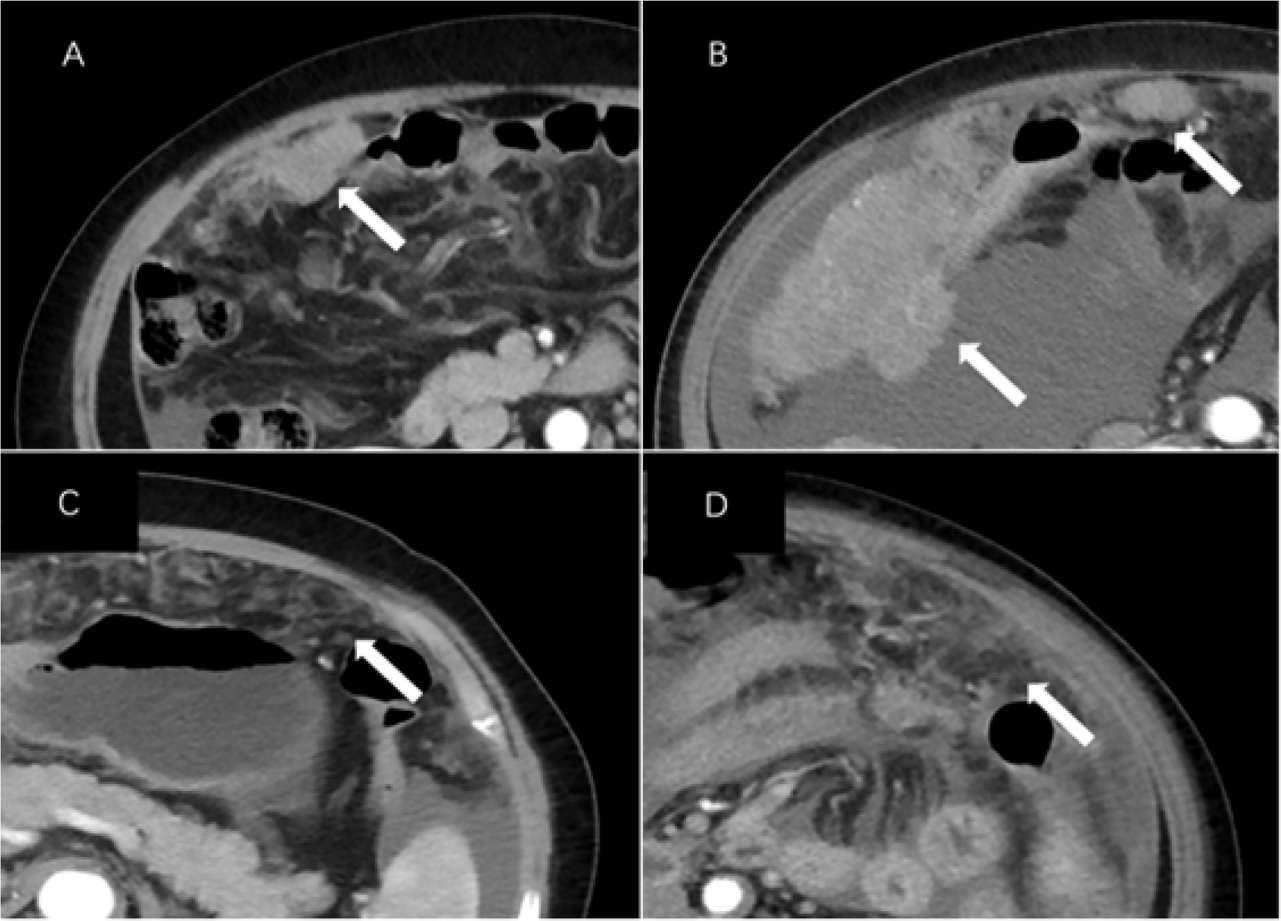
CT images of peritoneal metastases in a patient with epithelial ovarian cancer. Axial CT images of (A) a 66-year-old woman and (B) a 76-year-old woman with BRCA mutations (stage III and IV, respectively) showing nodular lesions (arrows). Axial CT images of (C) a 66-year-old woman and (D) a 68-year-old woman with invasive cancer and wild-type BRCA (both stage III, arrows).
